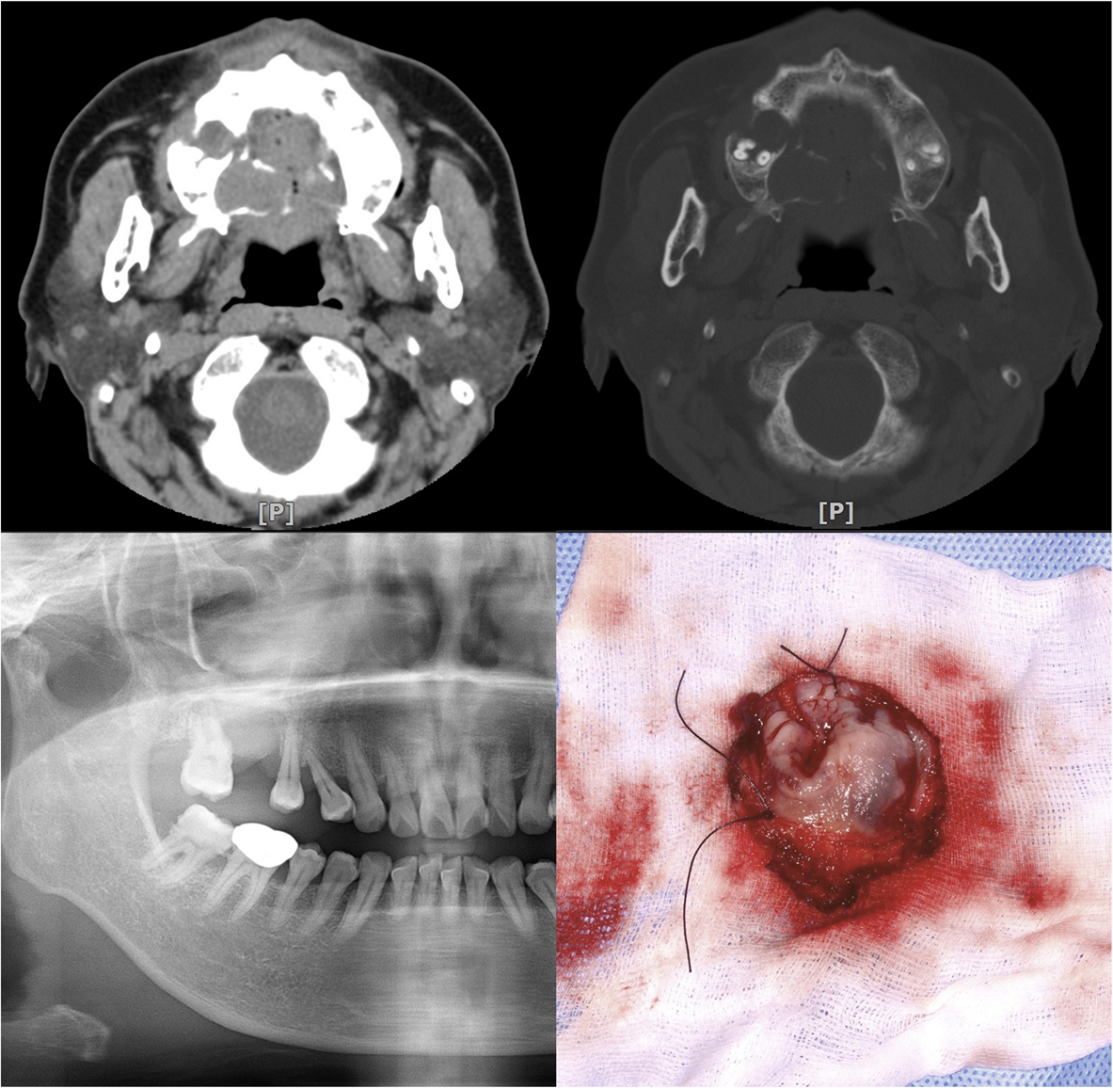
Preoperative computerized tomographic (CT) scan (*top*) and panoramic radiograph (*bottom left*) showed a round mass on the right palatal area. Postoperatively excised mass (*bottom right*)
